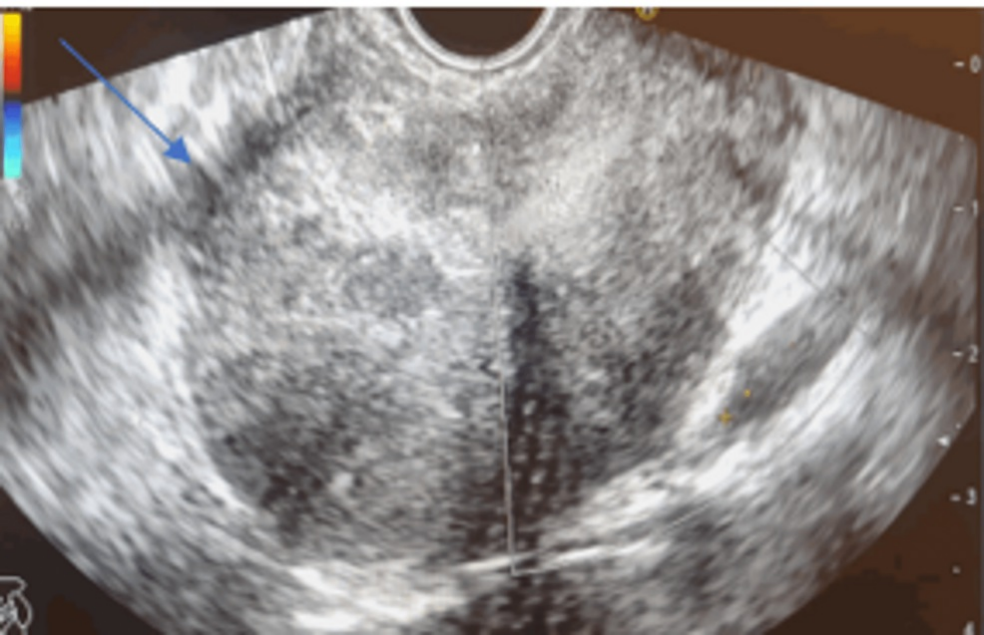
EUS image shows a well-defined gastric mass (5x5 cm) with irregular borders and heterogenous echogenicity arising from the fourth layer (arrow) EUS - endoscopic ultrasound
